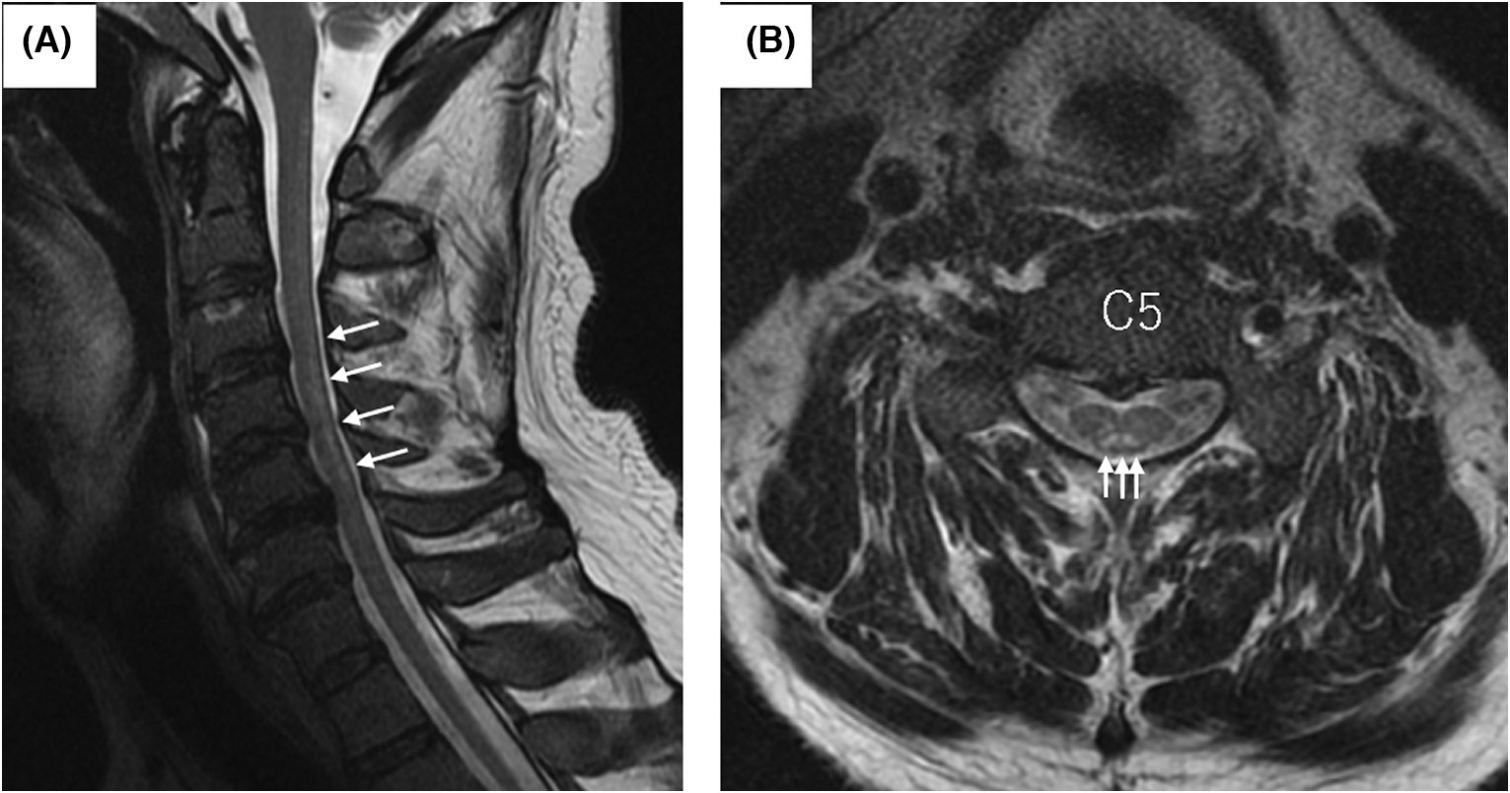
Magnetic resonance imaging of the cervical spine before treatment with subacute combined degeneration. (A) Sagittal T2WI showing longitudinally extensive hyperintense signal changes (white arrows) within the spinal cord. (B) Axial T2WI showing a bilateral hyperintense signal within the posterior funiculus, demonstrating the “inverted V‐sign” (white arrows).
